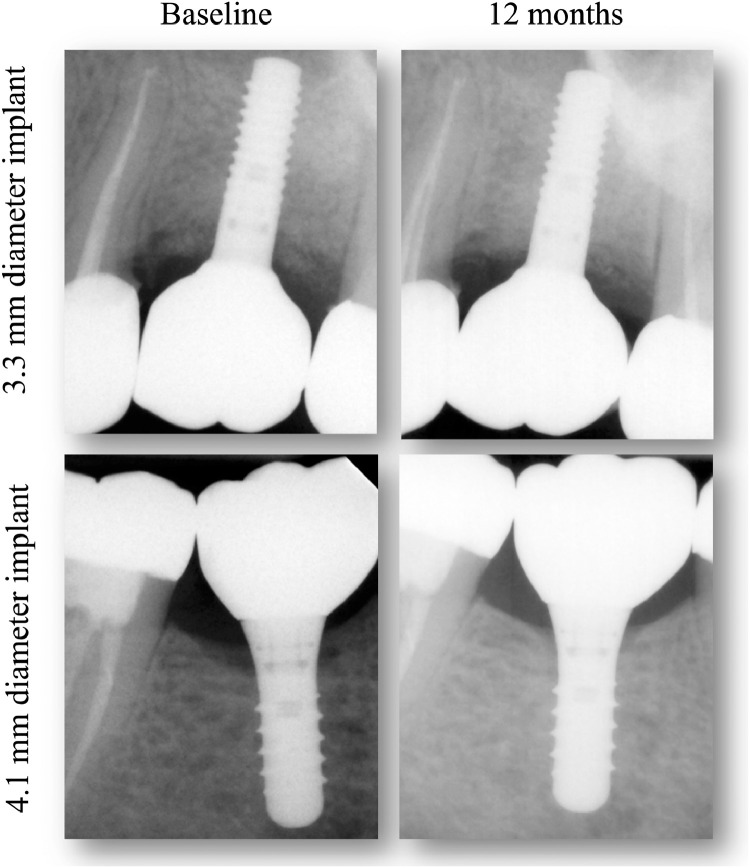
Radiographic assessment of marginal bone levels around narrow and standard diameter implants supporting single screw-retained monolithic zirconia crowns
